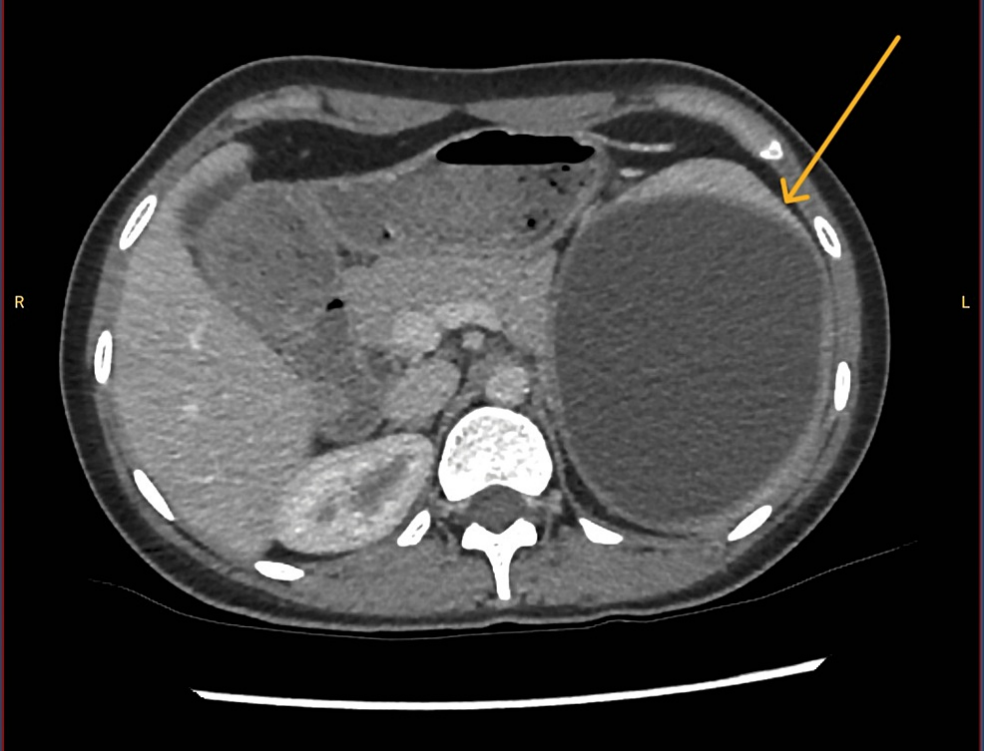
Axial view of CT portal venous showing the size of the isolated splenic hydatid cyst and associated mass effect Arrow indicates a small proportion of the remaining splenic parenchyma, encasing a large cyst
